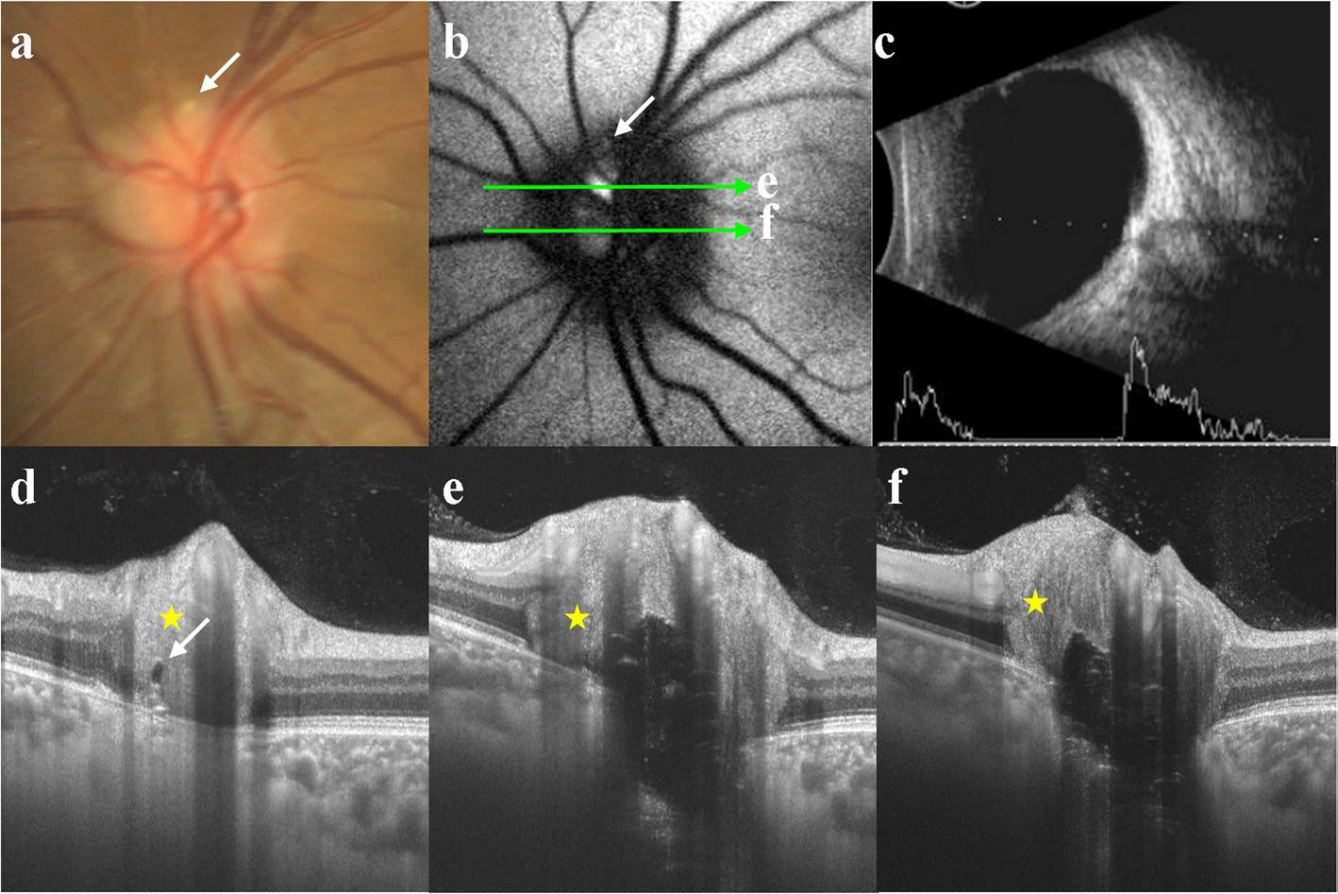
The relationship between ODD and AF with different depths a CFP shows pseudopapilledema and a punctate pale-yellow deposits (white arrow). b The weak autofluorescence (white arrow) corresponded to ODD in a and d (white arrows). Patchy autofluorescence was seen on the nasal side of the optic disc and the scan lines e and f corresponded to figures e and f, respectively. ODD in e was closer to the surface of optic disc than the ODD in f. Autofluorescence was brighter in e but weaker in f. c B-scan ultrasonography showed strong echo spot with acoustic shadowing. The yellow stars in d, e and f were PHOMS, corresponding to pseudopapilledema. CFP = color fundus photography; FAF = fundus autofluorescence; PHOMS = peripapillary hyperreflective ovoid mass-like structure
